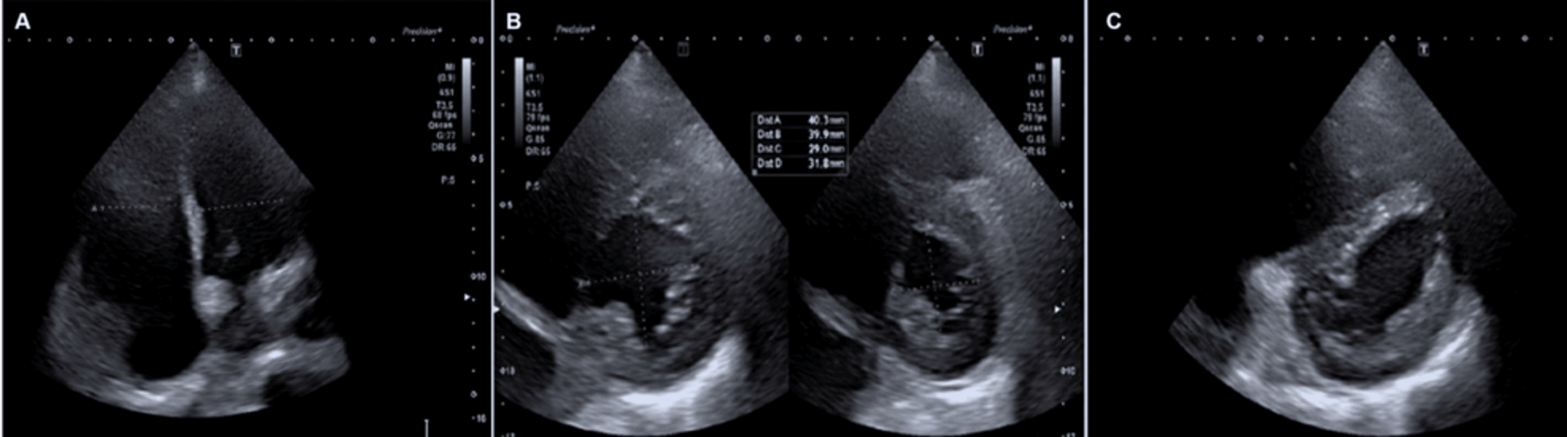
Transthoracic echocardiography (A) Apical four-chamber view demonstrating right ventricular dilation, with a right ventricle-to-left ventricle (RV/LV) ratio > 1. (B) Parasternal short-axis view showing interventricular septal displacement with reduced anteroposterior diameter of the left ventricle. (C) "D-sign" observed during systole, reflecting right ventricular pressure overload and septal flattening.
